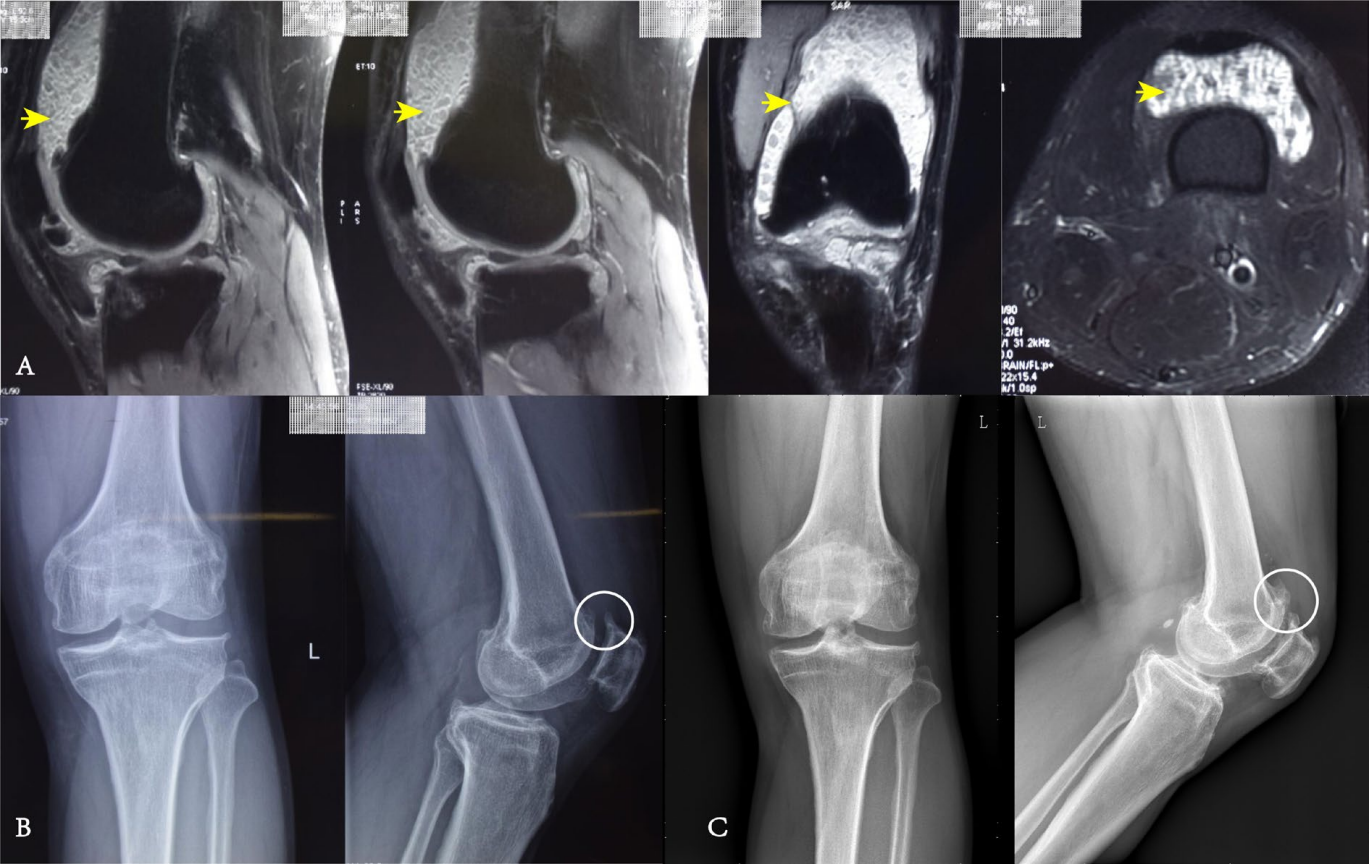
The patient is a 53‐year‐old male who presented with left knee pain accompanied by locking for 6 years. Physical examination revealed tenderness in the left knee, significant patellar crepitus, and marked limitation of movement. During surgery, mild synovial hyperplasia was observed, along with obvious degeneration of the articular cartilage on the femoral condyles and patella. Several hard, variably sized loose bodies were found within the joint space. Pathological examination showed bone/cartilage components consistent with synovial chondromatosis. Postoperatively, the patient's symptoms improved significantly, and his function recovered well. (A) The patient's MRI shows multiple loose bodies within the knee joint; (B) the patient's X‐ray reveals secondary osteoarthritis in the knee joint, with significant osteophyte formation in the patellofemoral joint; (C) the patient's X‐ray at the last follow‐up. The yellow arrows indicate the loose bodies in the suprapatellar pouch of the knee, and the white circles mark the osteophytes.
